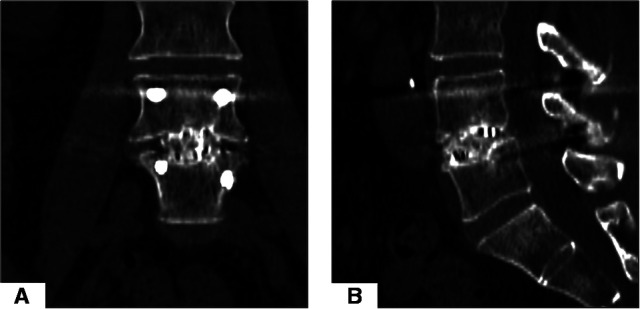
CT scan for three months after surgery. (A) Coronary scanning. (B) Sagittal scanning.
